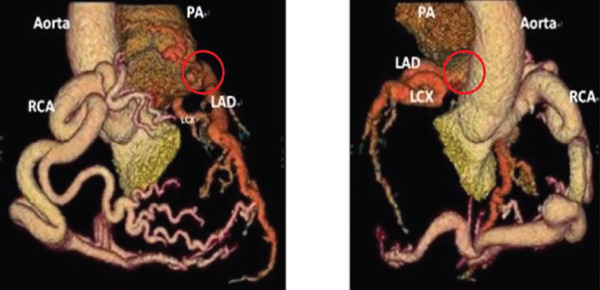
Coronary CTA: front view (left) and rear right view (right). RCA: right coronary artery; PA: pulmonary artery; LCX: left circumflex; LAD: left anterior descending artery. The red circle indicates the origin of the left coronary artery from the pulmonary artery.
